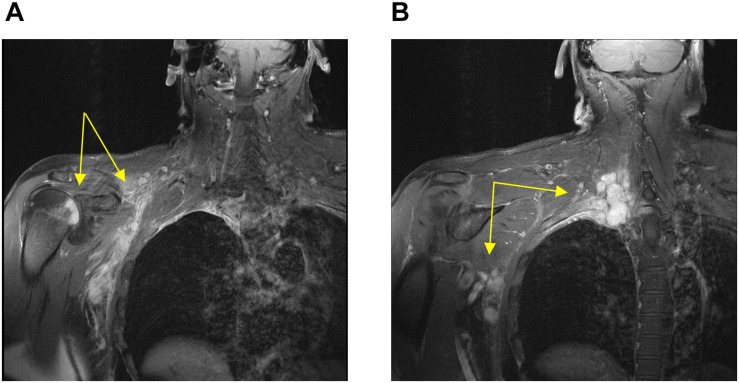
Magnetic resonance imaging conducted at first diagnosis showing soft tissue-masses (yellow arrows) with infiltration of the right humerus (A) and the right pleura (B).
